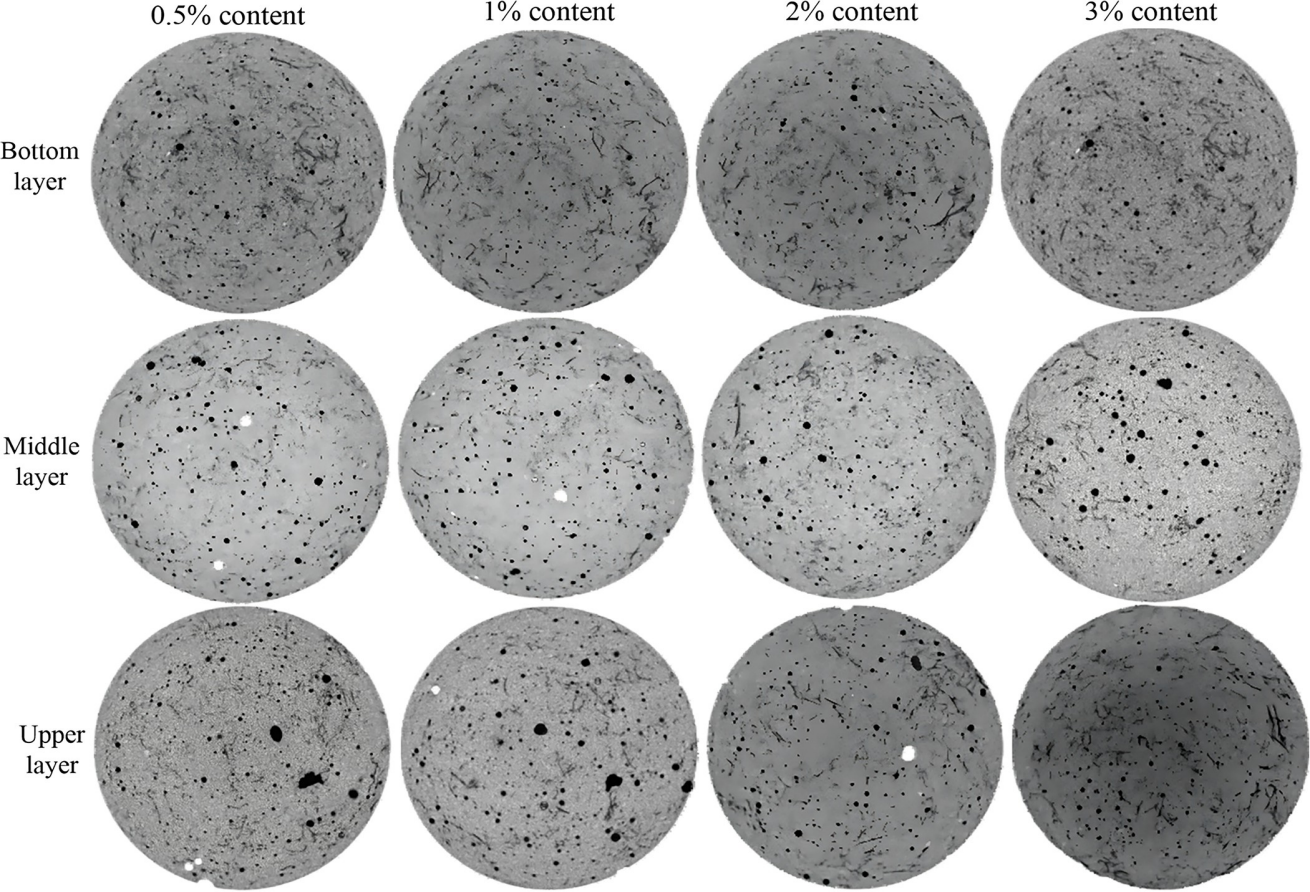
CT scanning of cement mortar with different content of luffa fiber.
